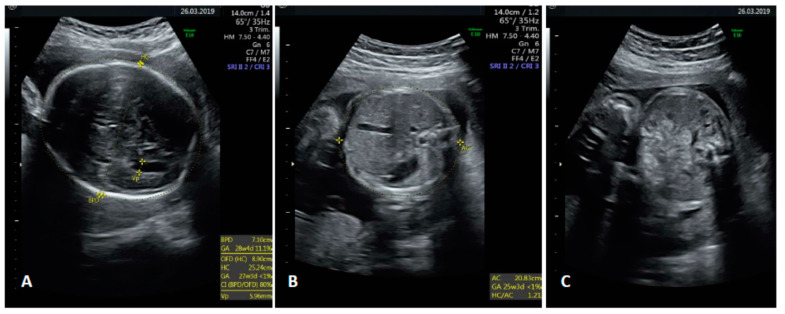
Fetal ultrasound findings for Case 2 at 30 + 3 weeks showing a normal brain in the transventricular plane (A), normal abdomen (B) and minor bowel echogenicity, consistent with the diagnosis of early onset fetal growth restriction (C). Neither hepatosplenomegaly nor other signs suggestive of fetal infection were detectable at that stage.
